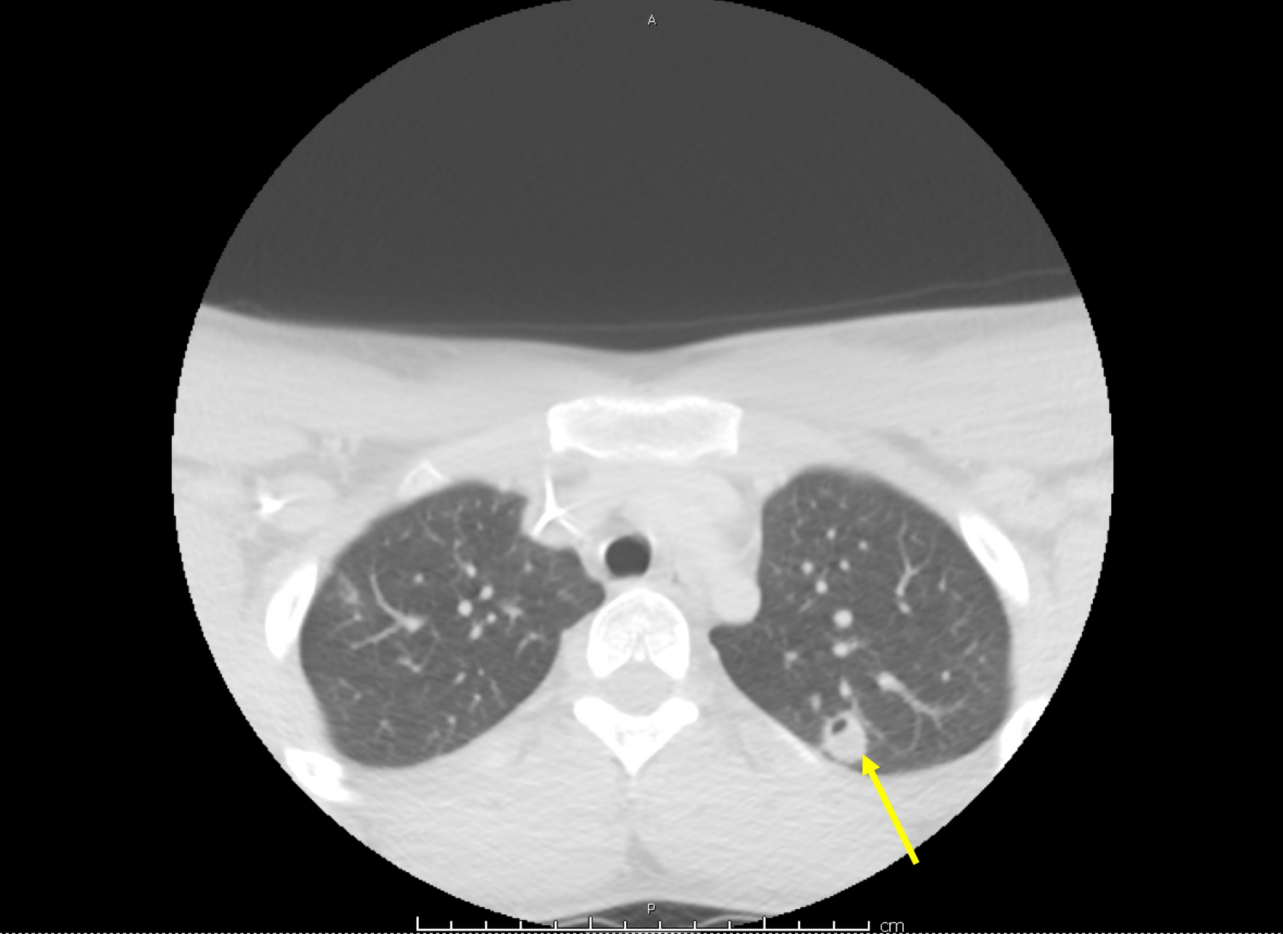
Chest computed tomography with contrast. Interval development of cavitation was seen later in nodular infiltrates consistent with septic emboli.
